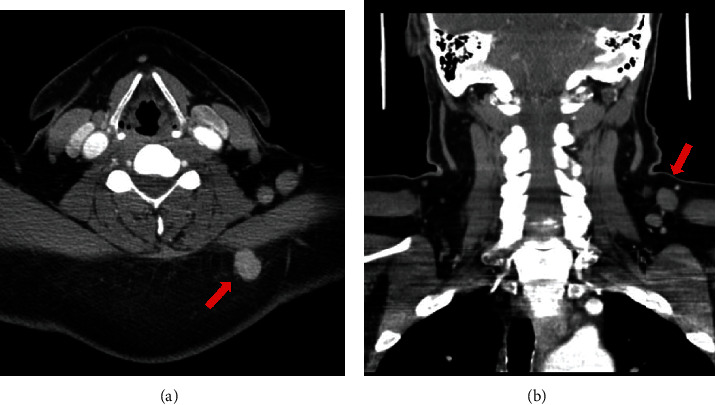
(a, axial view) CT scan with contrast demonstrated a hyperdense rounded mass in the subcutaneous tissue of the left side of the neck, posterior to the trapezius muscle, corresponding to the patient's palpable primary mass (red arrow). (b, coronal view) Several lymph nodes (red arrow) in the posterior triangle anterior to the trapezius muscle ranging in size up to 1.5 cm in diameter were demonstrated on CT scan.
